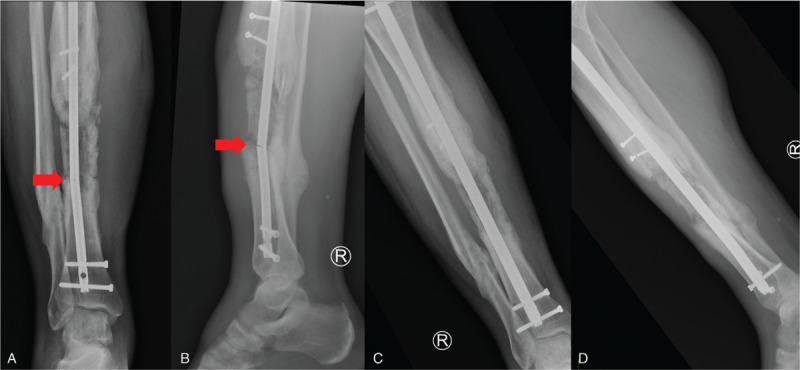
Tibial shaft nonunion with broken IM nail. Radiographs of 34-year-old male 6 months after receiving bone grafting of a large bone defect following the induced membrane (Masquelet) technique for an open tibial fracture (A and B). He unfortunately broke his tibial nail prior to his bone graft consolidating fully. Postoperative radiographs removal of the broken nail and exchange to a larger tibial nail (C and D). The broken nail was removed using a commercial extraction hook.
